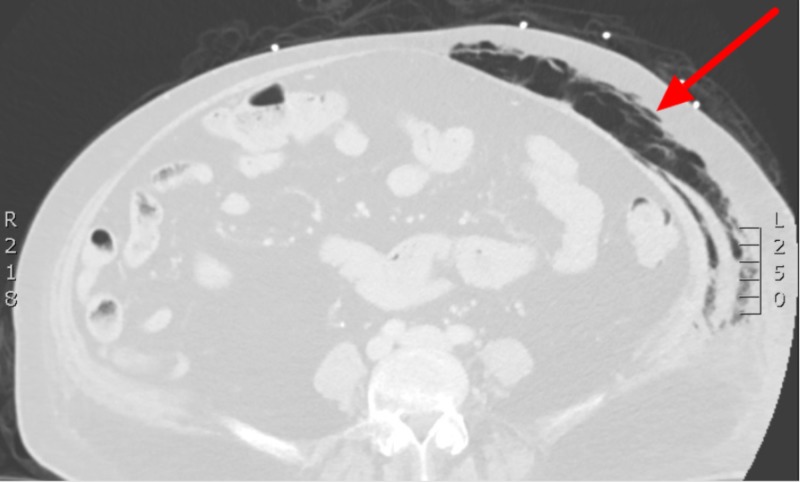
Transverse CT image showing soft tissue gas (red arrow) in the left anterior abdominal wall.
